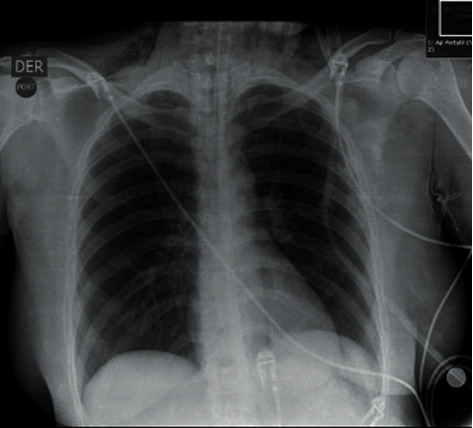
Chest X-ray: emphysema is observed in the cervical region, the cardiothoracic index was within normal limits, and no rib fractures, pneumothorax, or pleural effusion are identified, without consolidations or interstitial infiltrate.
